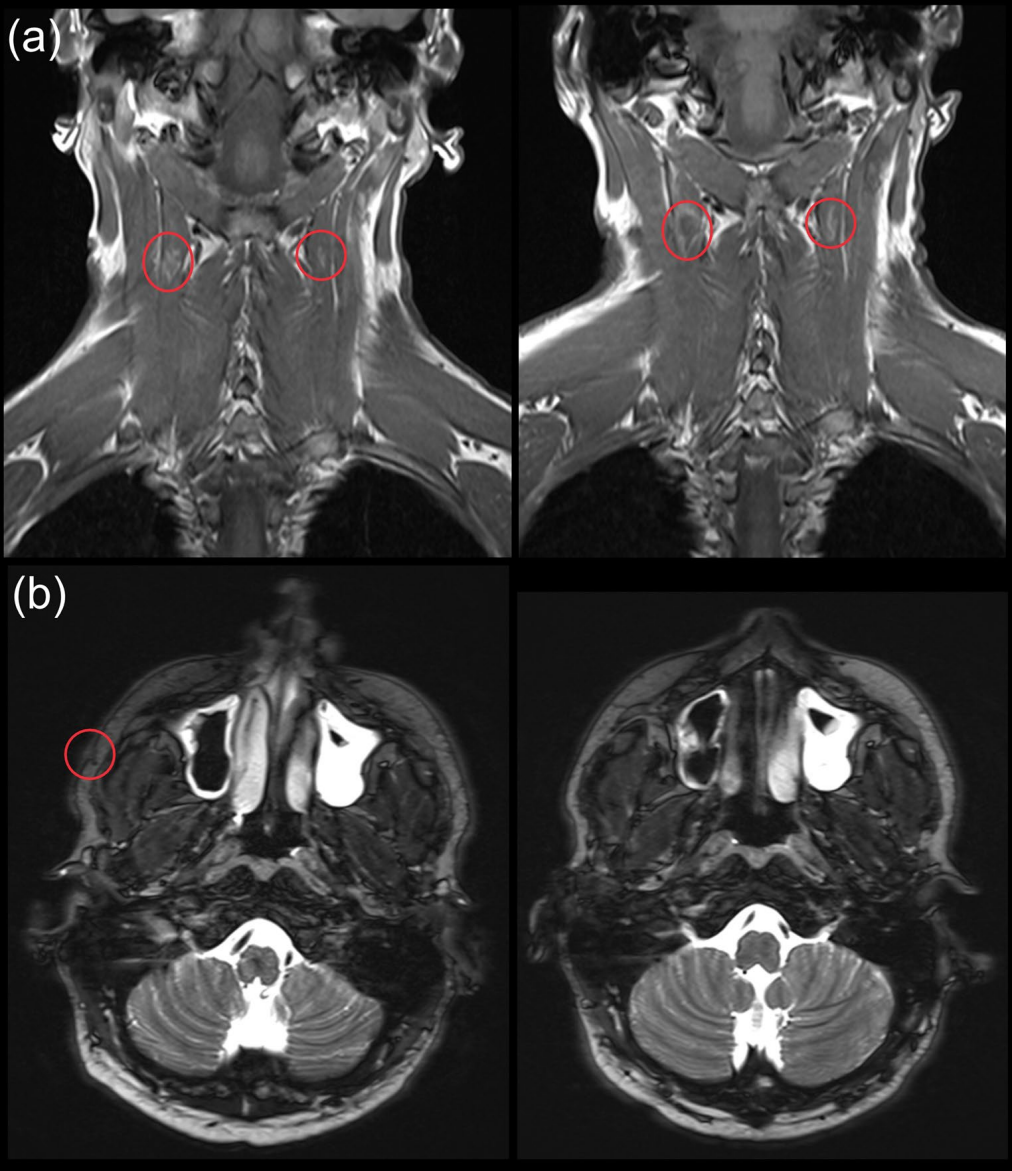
Exemplary MRI data. (a) Living strangulation victim showing MRI muscle findings in the coronal T_1_-weighted TSE sequence (red circles). Left: MRI scan acquired within timeframe 1 (4 days) after the incident, the muscle findings were rated as hemorrhages. Right: MRI scan acquired within timeframe 2 (11 days) after the incident, the muscle findings were still present and rated as edema. (b) Living strangulation victim showing an edema of the cutis in the transversal T_2_-weighted TSE DIXON opposed-phase MRI data (red circle). Left: MRI scan acquired within timeframe 1 (3 days) after the incident, the finding in the cutis was rated as edema. Right: MRI scan acquired within timeframe 2 (9 days) after the incident, the finding was no longer present (no red circle)
